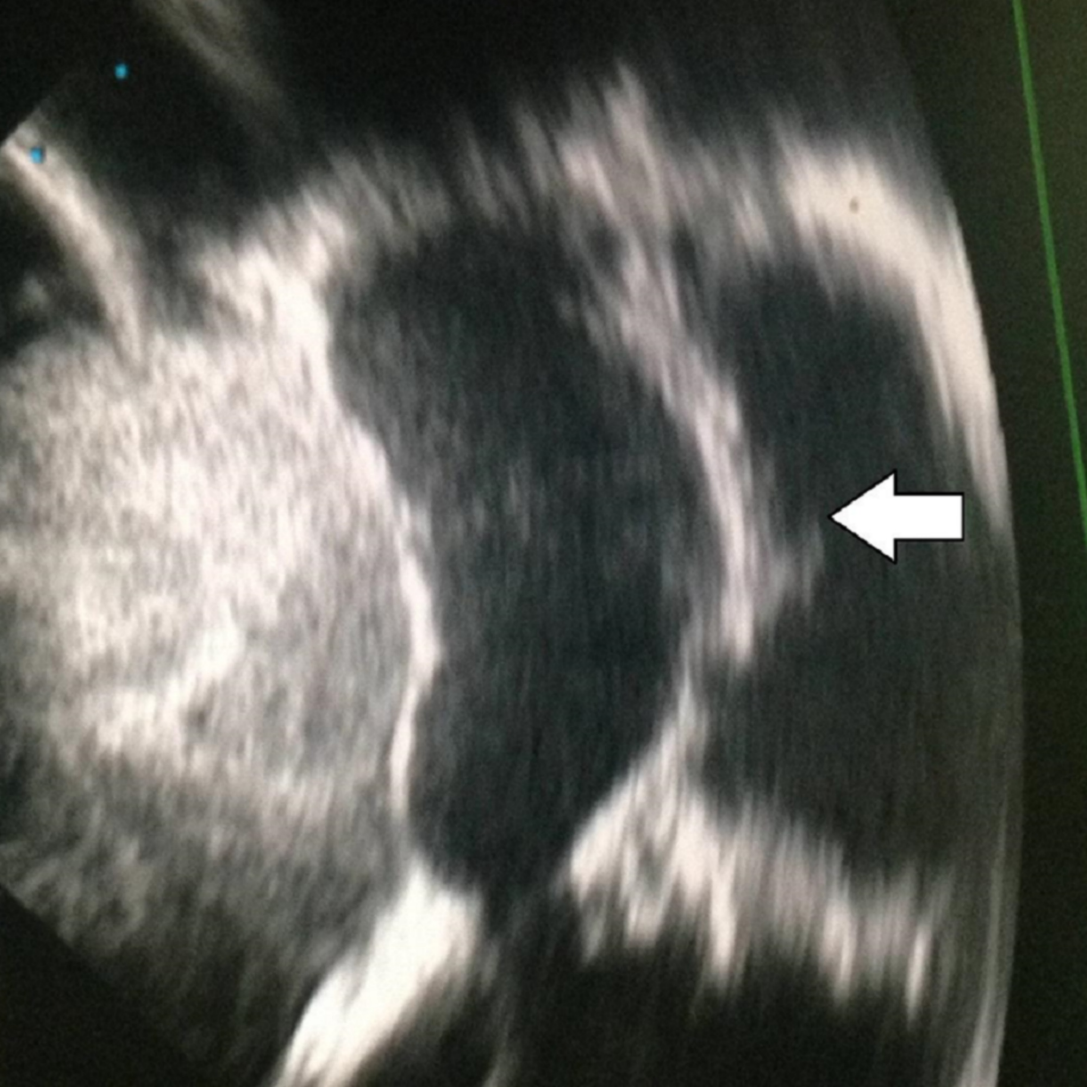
Echocardiographic image showing the transverse band in the left ventricular chamber
